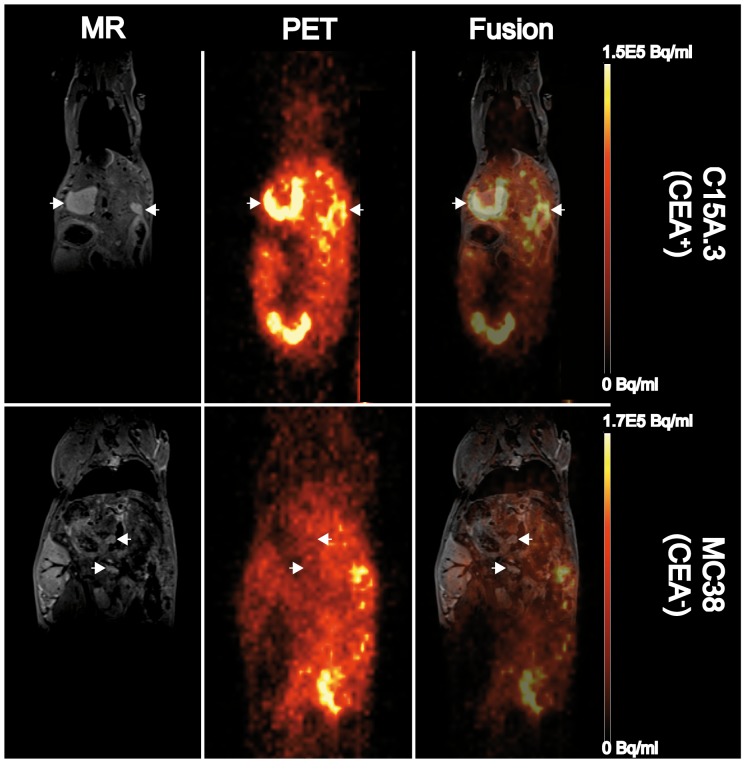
MRI and PET scan results for liver metastases originating from C15A.3 and MC38 cells 24 h post-injection of ^64^Cu-DOTA-M5A antibody. MRI images clearly show the location of the liver metastases (arrows) and were used to draw regions of interest (ROIs) for evaluating the PET data. Immuno-PET images indicate strong signals in the areas of the CEA-positive C15A.3-derived liver metastases. No enhanced tracer uptake was observed in the areas of CEA-negative MC38 derived metastases.
